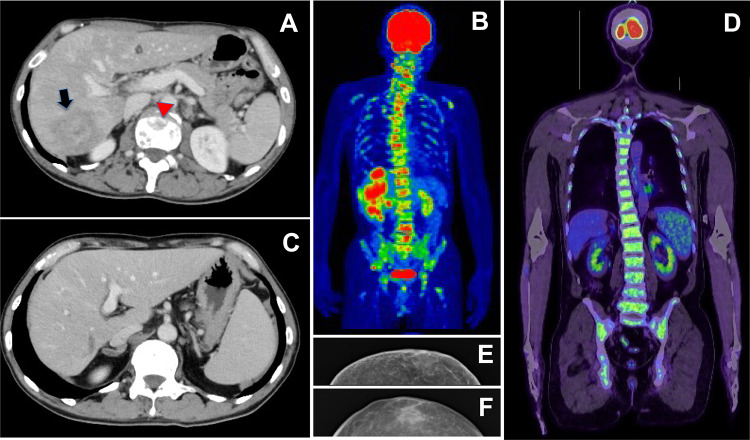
Patient images. A. CT image at the initial examination. The black arrow indicates liver metastasis, and the red arrowhead indicates bone metastases. B. PET-CT image before chemotherapy. The regions with higher uptake of 18FDG signal are indicated in the liver and whole spine. C. CT image after five cycles of CBDCA + PTX treatment. Liver metastasis disappeared and the spotty osteolytic region was repaired. D. PET-CT image after eight cycles of CBDCA + PTX treatment. The uptake in the liver disappeared and the spine signals decreased. E. Mammography of the right breast. F. Mammography of the left breast. No tumour was detected in both breasts
